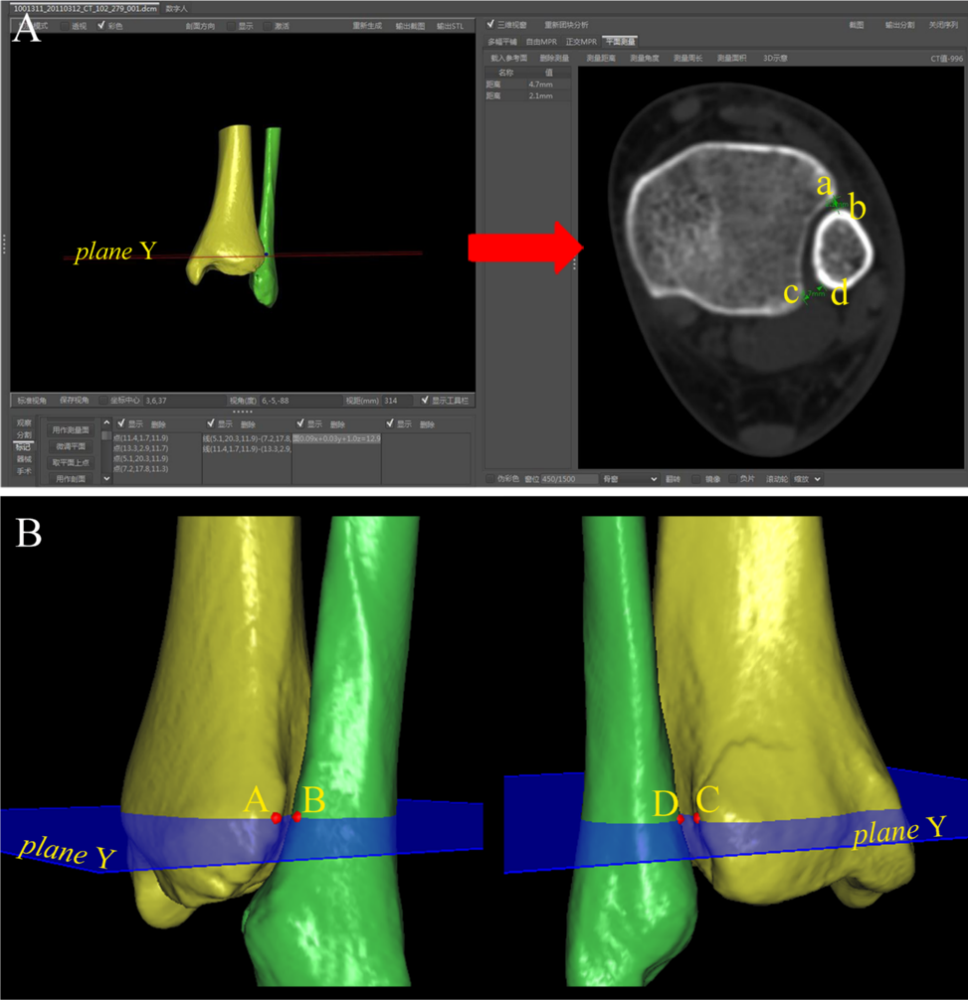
The tibiofibular clear space was measured in the 2-D axial and 3-D images of CT scans at same level of plane Y (red arrow). a TCS-A = anterior tibiofibular clear space (line ab); TCS-P = posterior tibiofibular clear space (line cd). b TCS-A (line AB) and TCS-P (line CD) in 3-D images were measured
